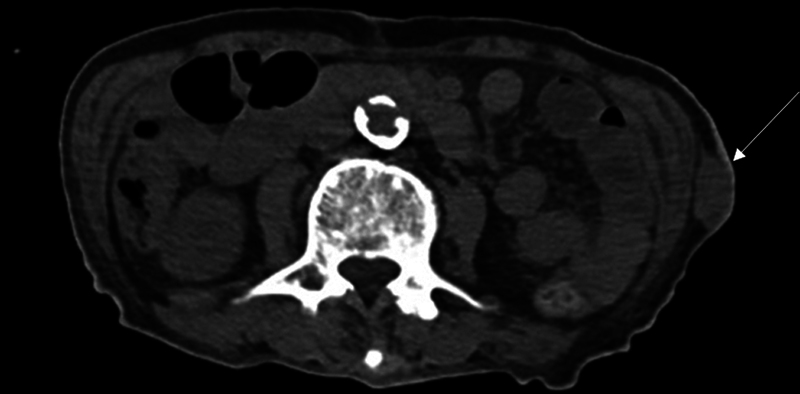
Computed tomography scans revealed a huge mass measuring 4.5 × 2.2 cm in the flank, with reports suggesting possible malignancy.
